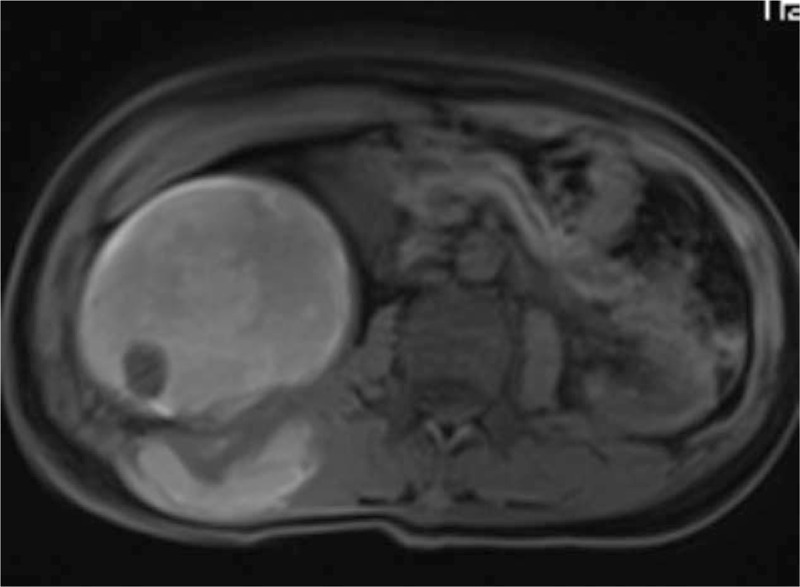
MRI: The nodular of wall showed equal or slightly lower signals. However, most of the cystic area revealed equal or slightly high signals.
